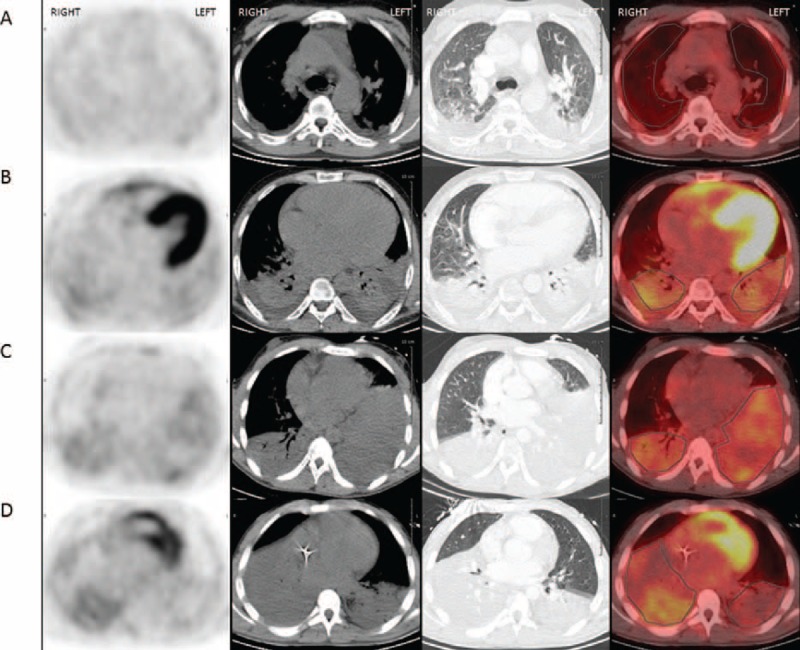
Examples of transaxial cross-section ^18^F-FDG PET CT images from 3 patients with sickle cell acute chest syndrome. From left to right, PET scan, CT scan with mediastinal and parenchymal windows, and PET CT fusion image. Cross-section images show low ^18^F-FDG uptake in normally aerated upper lobes (A) and higher ^18^F-FDG uptake in consolidated lower lobes (B) of the same patient (a bilateral pleural effusion is not included in the ROIs) and high ^18^F-FDG uptake in consolidated lower lobes of 2 distinct patients (C, D) with normally aerated adjacent lung parenchyma. CT = computed tomography, ^18^F-FDG = ^18^F-fluorodeoxyglucose, PET = positron emission tomography, ROIs = regions of interest.
